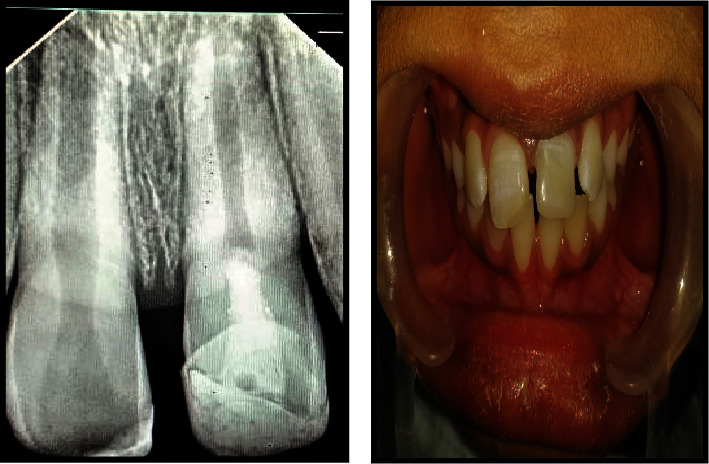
Radiograph demonstrating the faster progression of apical closure on tooth #21 with MTA than tooth #11 after 2 years. The patient had no symptoms. Cvek's stage 5.
